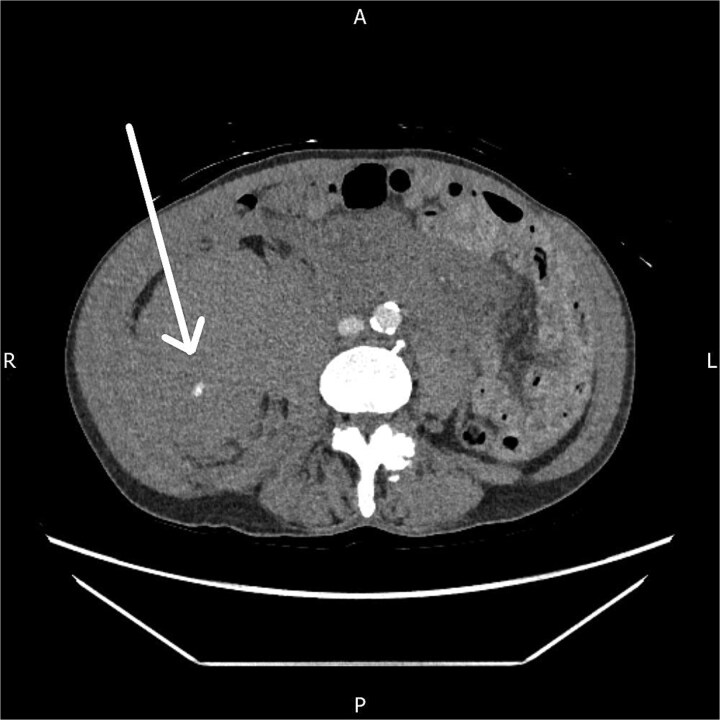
Axial CT angiogram showing persistent right-sided retroperitoneal haematoma with ongoing active contrast extravasation.
